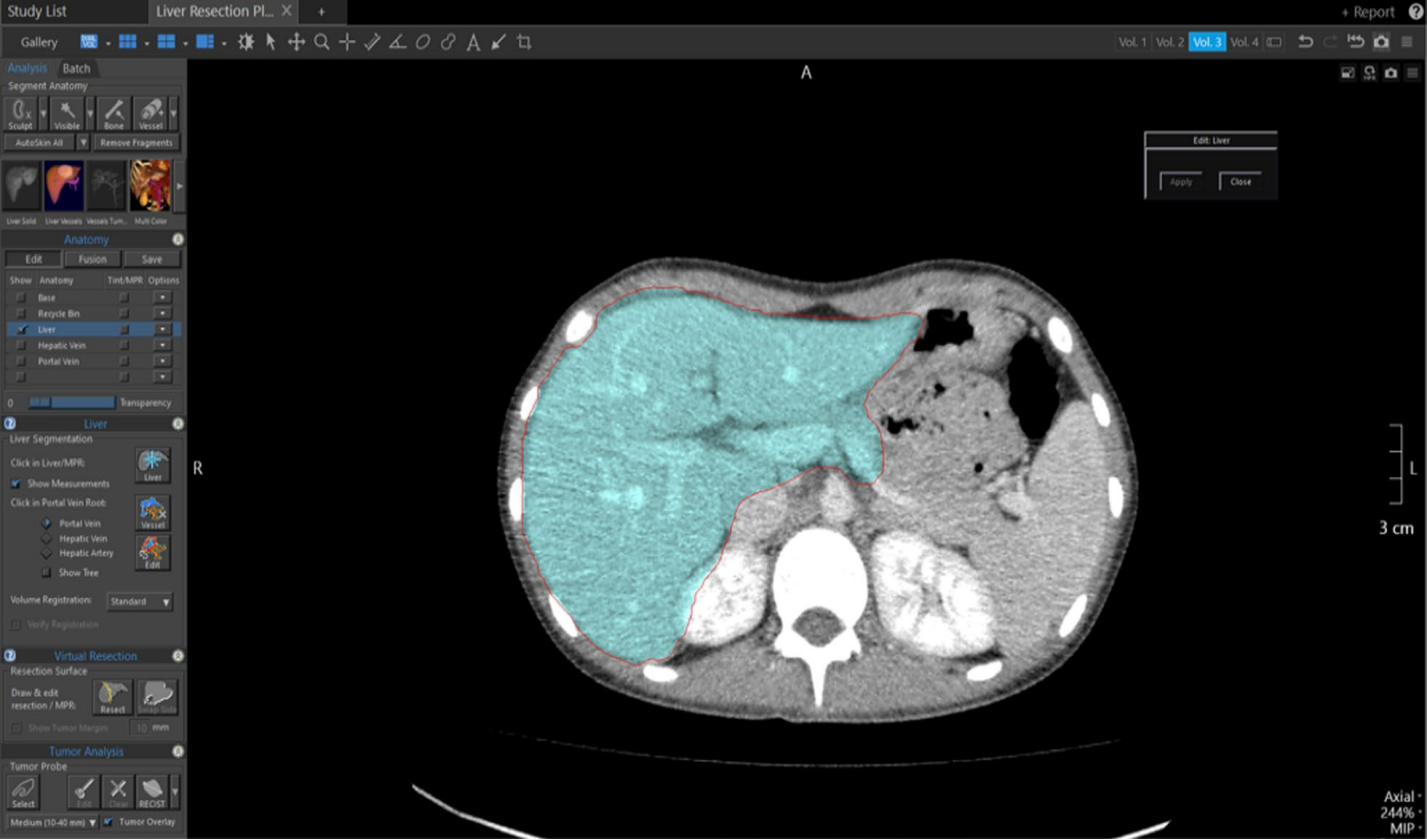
False-positive result acquired by CT liver analysis application. The liver parenchyma automatically generated by the software (blue color) includes parenchyma of the right kidney and pancreas
